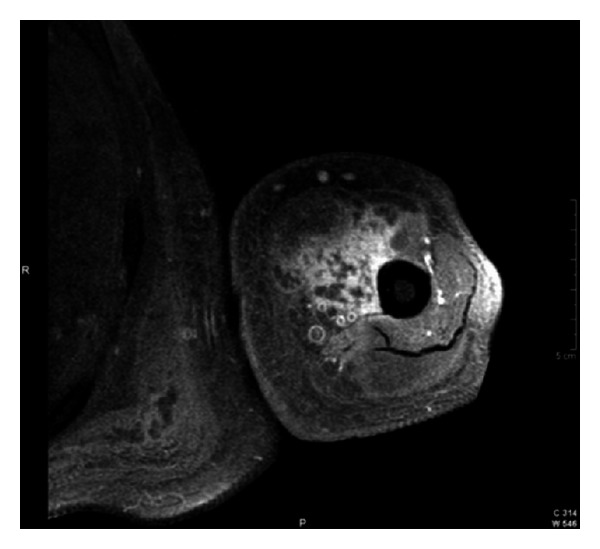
Left upper extremity MRI. T1 weighted image. Heterogenous patchy contrast enhancement consistent with myonecrosis.
